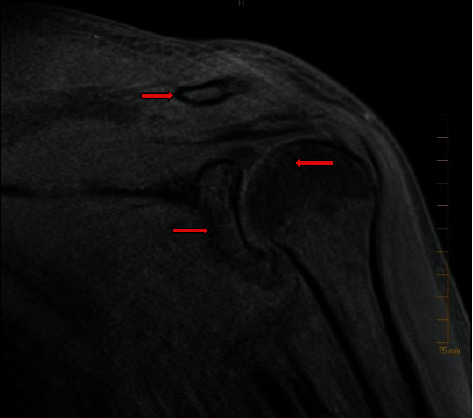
MRI left shoulder revealed acromioclavicular joint synovitis with periarticular soft tissue edema consistent with septic arthritis and distal clavicle marrow edema suggestive of osteomyelitis. A subcentimeter abscess in the subacromial bursa was also noted.
